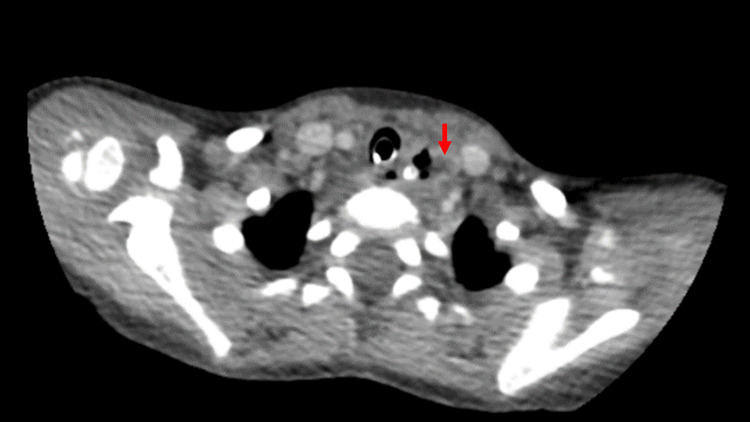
CT of the neck and chest Two days later, newly developed compression of the medial wall of the left common carotid artery caused significant luminal narrowing with intraluminal thrombus (red arrow) at the level of the T1. Here, the carotid-esophageal fistula cannot be totally excluded. The distal aspect of the left common carotid artery and the remaining major vasculature are patent. CT: computed tomography
